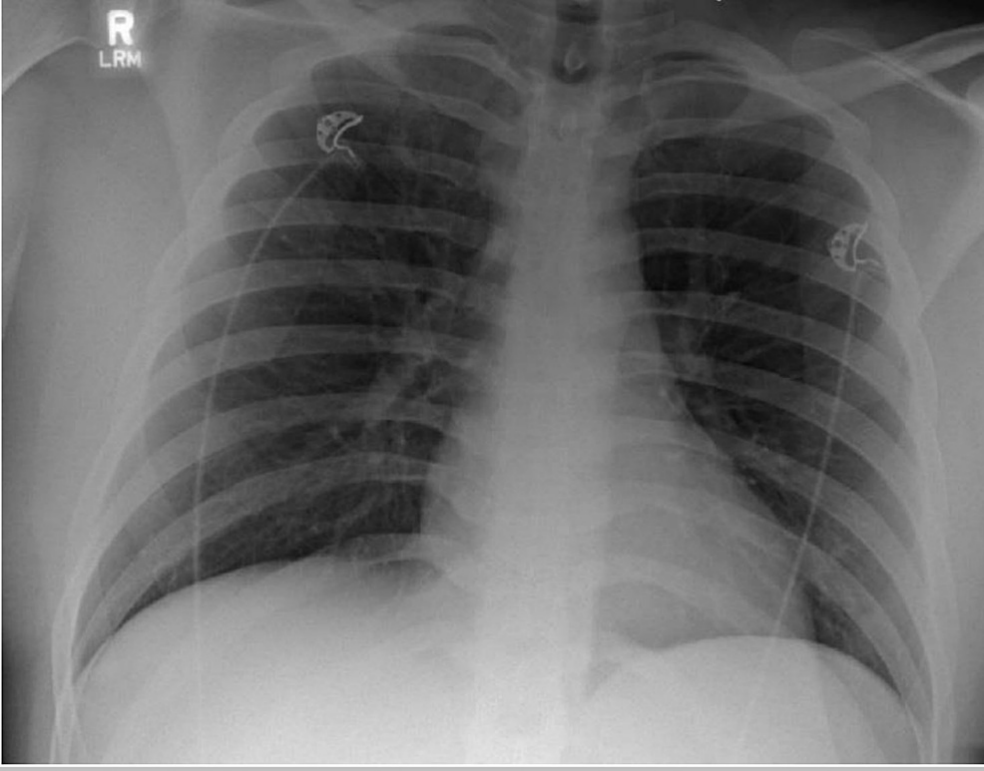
Initial chest X-ray upon presentation.
